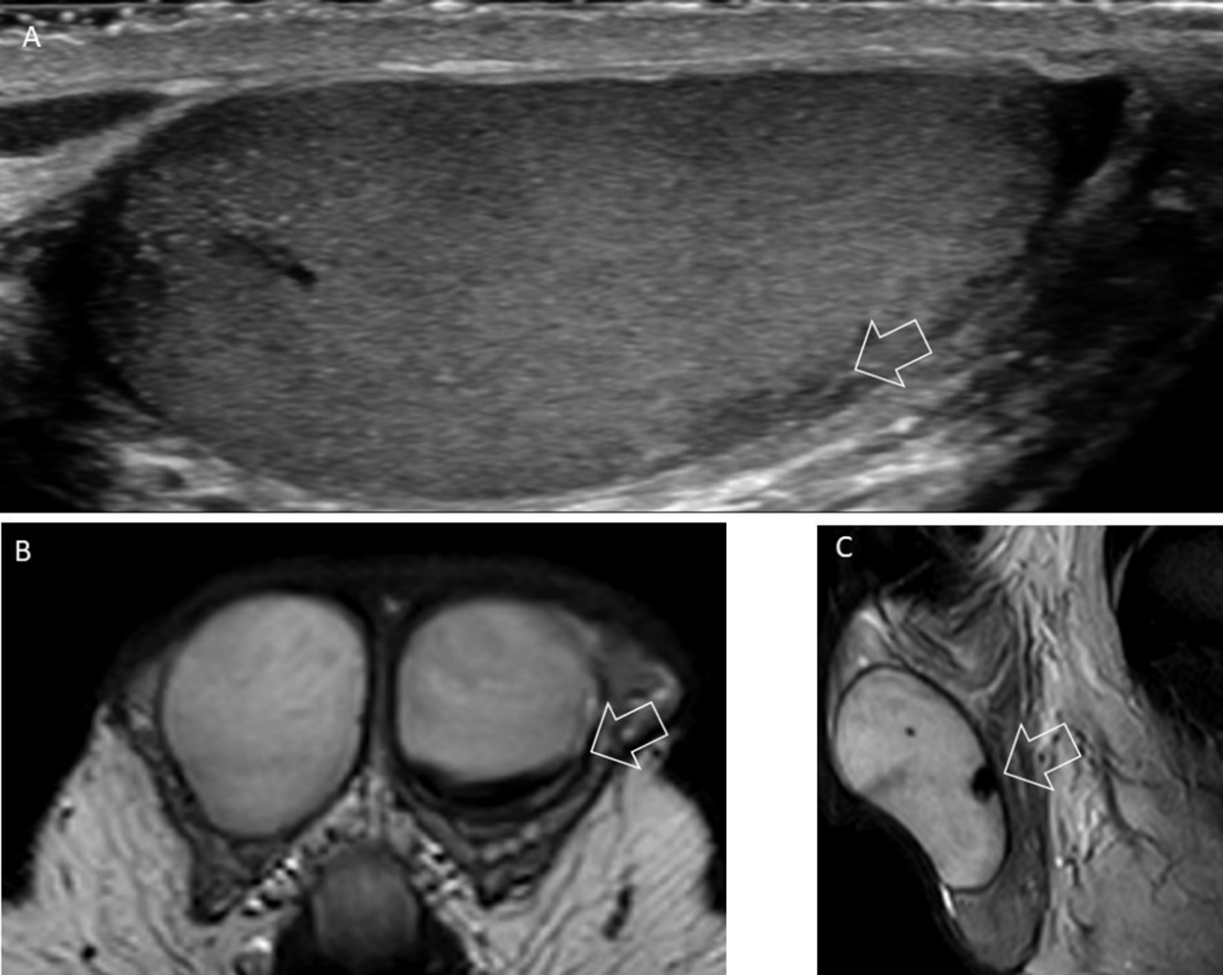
(A) Last follow up ultrasound showed a small residual hypoechoic area (arrow). Axial (B) and sagittal T2‑weighted (C) images showed a newly appearing hypointense signal of the lesion corresponding to hemosiderin deposition (arrow).
